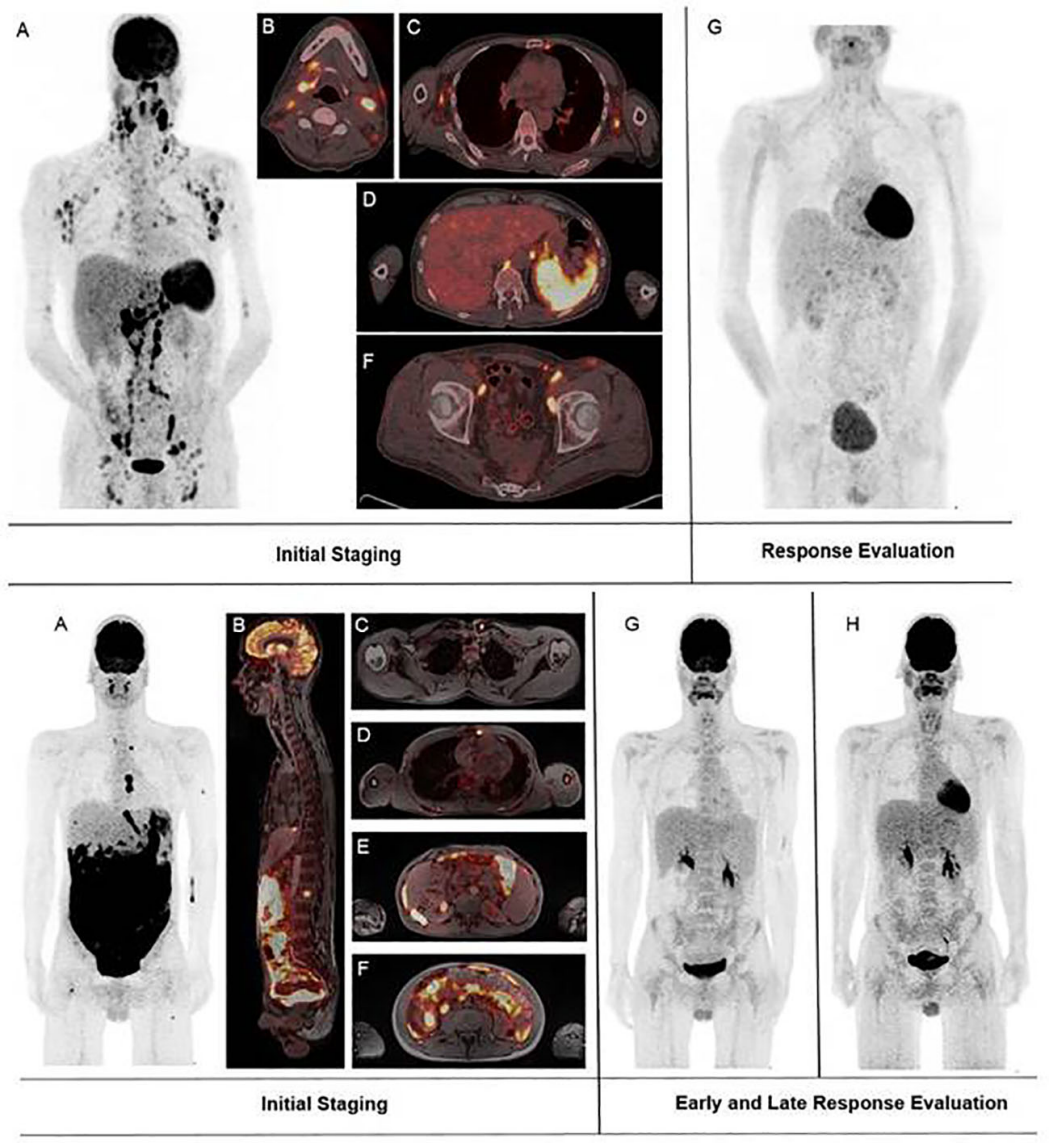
Representative fluorodeoxyglucose positron emission tomography–computed tomography and magnetic resonance tomography (^18^F-FDG PET/CT and MRT) images of two patients who achieved a complete response. Upper panel: (A–F)
